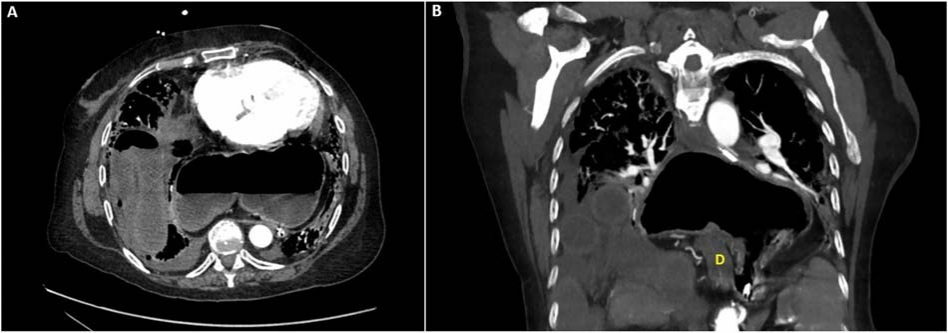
Computed tomography of the chest and abdomen: (A) axial section demonstrating incarcerated small bowel within the right hemithorax as well as stomach within the posterior mediastinum. (B) Coronal section demonstrating incarcerated small bowel within the right hemithorax above the liver as well as hiatal hernia containing stomach in an organoaxial rotation as well as the duodenum (D).
